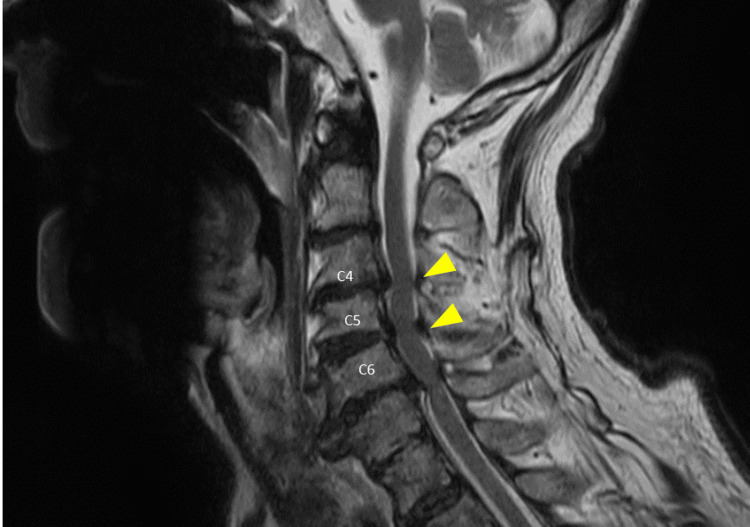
Cervical magnetic resonance imaging on initial evaluation On T2-weighted imaging, no cervical fracture or epidural hematoma was identified, and no definite intramedullary signal abnormality was observed. However, sagittal canal narrowing was noted at the C4/5 and C5/6 levels.
